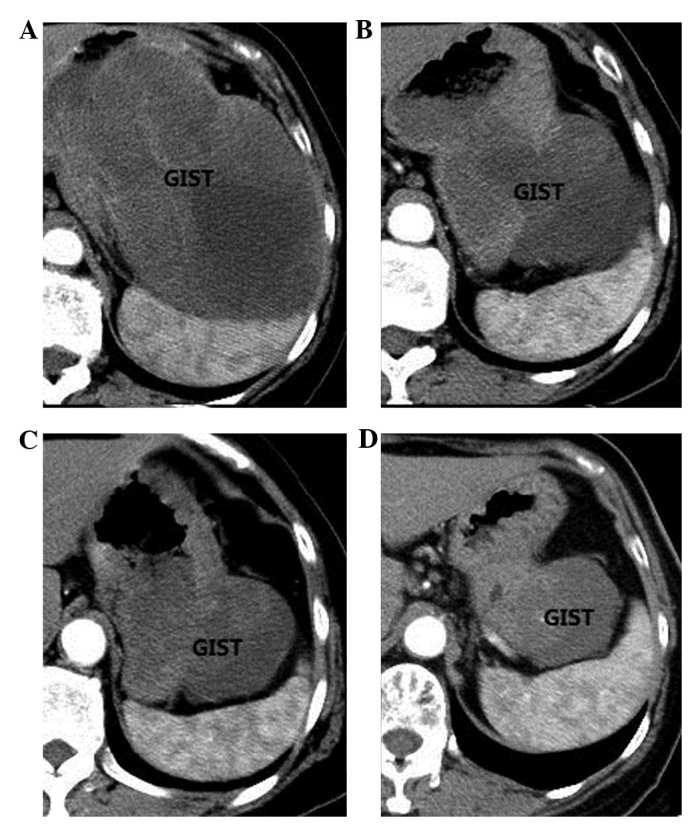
Enhanced CT scan of GIST (A) before imatinib treatment, and (B) 2, (C) 4 and (D) 6 months after therapy revealed a significant decrease in the tumor size. CT, computed tomography; GIST, gastrointestinal stromal tumor.
